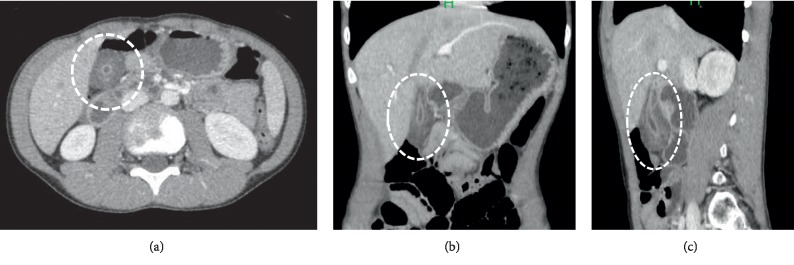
Abdominal contrast-enhanced computed tomography revealed a circular solid mass-like lesion (circle) near the normal-looking gallbladder on admission: (a) axial view, (b) coronal view, and (c) sagittal view.
